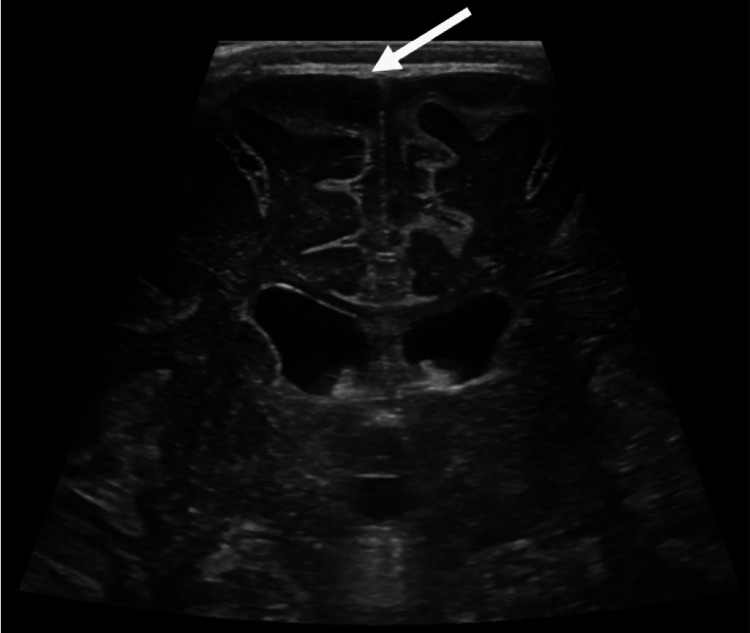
Head ultrasound, coronal mid view, demonstrates a prominent extra-axial fluid space (arrow).
